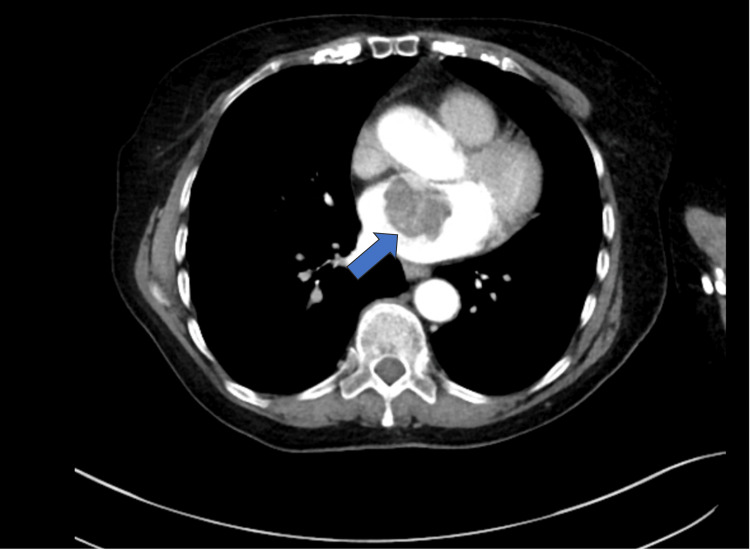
Chest computed tomography (CT) scan showing a heterogeneous mass within the left atrium (blue arrow) measuring 3.9x3.8x4.3 cm (anteroposterior, transverse, craniocaudal).
